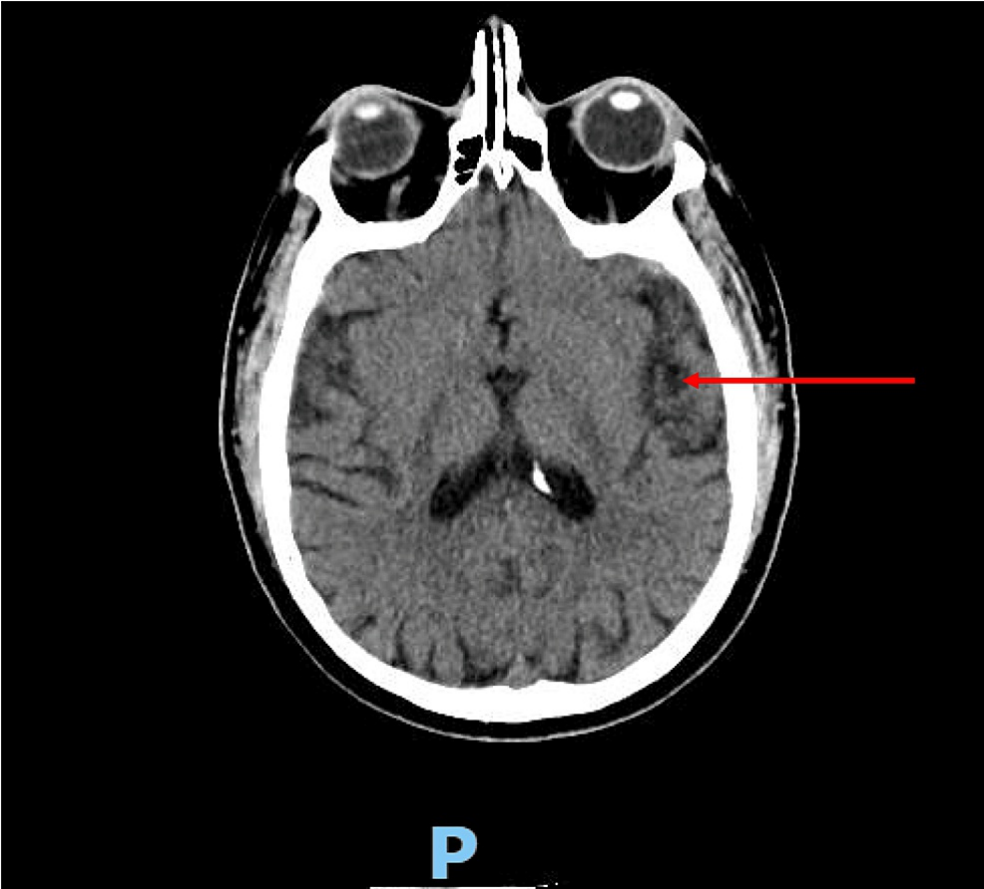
Brain computed tomography showing a focal low attenuation area representing septic emboli or meningoencephalitis.
